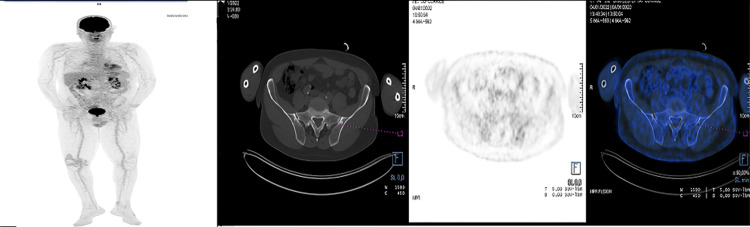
PET/CT three months after radiotherapy revealed a decrease in size and fixation of tumor hypermetabolic infiltration of the duodenum (SUVmax: 7.2 versus 16.8). Partial metabolic response to duodenal injury. The appearance of hypermetabolic bone lesions of the left iliac bone and the lower extremity of the left femur, without paramedullary extension PET/CT, positron emission tomography/computed tomography; SUVmax, maximum standardized uptake value
